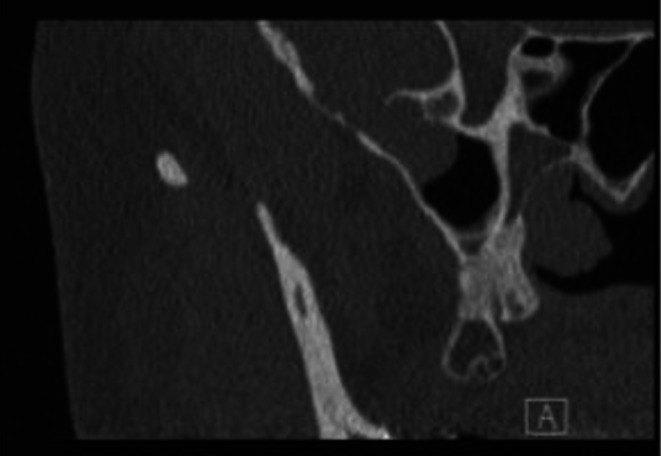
Right sphenoid sinus defect shown in coronal CT scan.
